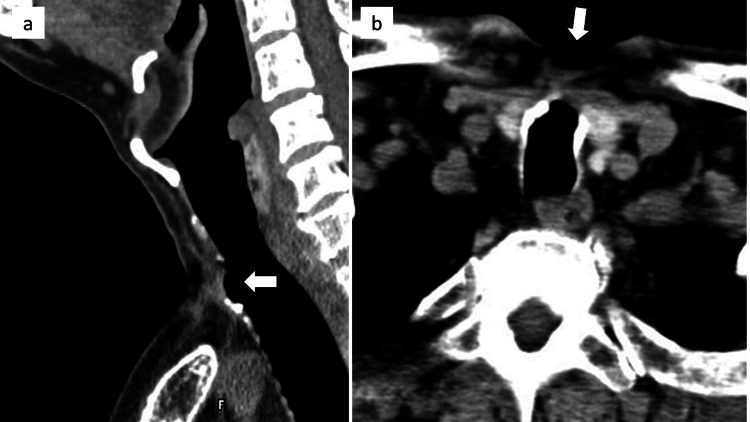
Postoperative computed tomography (CT): (a) sagittal view; (b) axial view. The tracheal cartilage defect was covered, and the amount of soft tissue reinforced by the sternocleidomastoid muscle was maintained.
